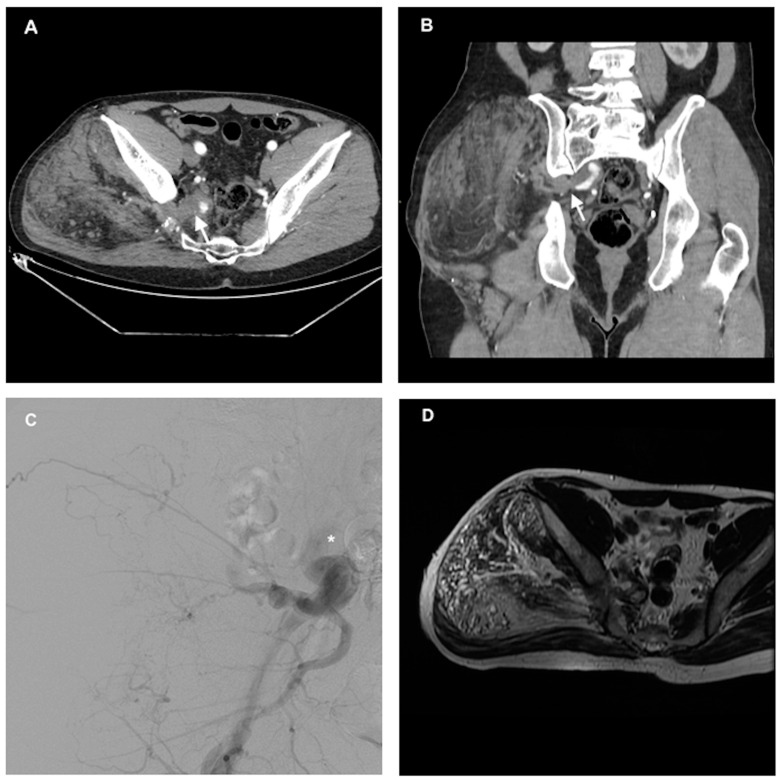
(A) Contrast-enhanced CT (axial) in the late arterial phase performed 6 months after the fourth (and last) embolization. The mass is considerably reduced in diameter, with some small arterial feeders, and thrombosis of the superior gluteal artery (arrow). (B) Coronal view demonstrating a great reduction in pathologic vascularization with a significant reduction in the caliber of the internal iliac artery (arrow). (C) DSA performed at the end of the fourth embolization procedure, with the tip of the catheter (Cobra 5 F) in the internal iliac artery (asterisk) demonstrating a great reduction in vascularization and a complete stop in the arterial flow in the superior gluteal artery. (D) Twelve-month follow-up MRI after the last embolization showing an axial T2 non-saturated sequence.
